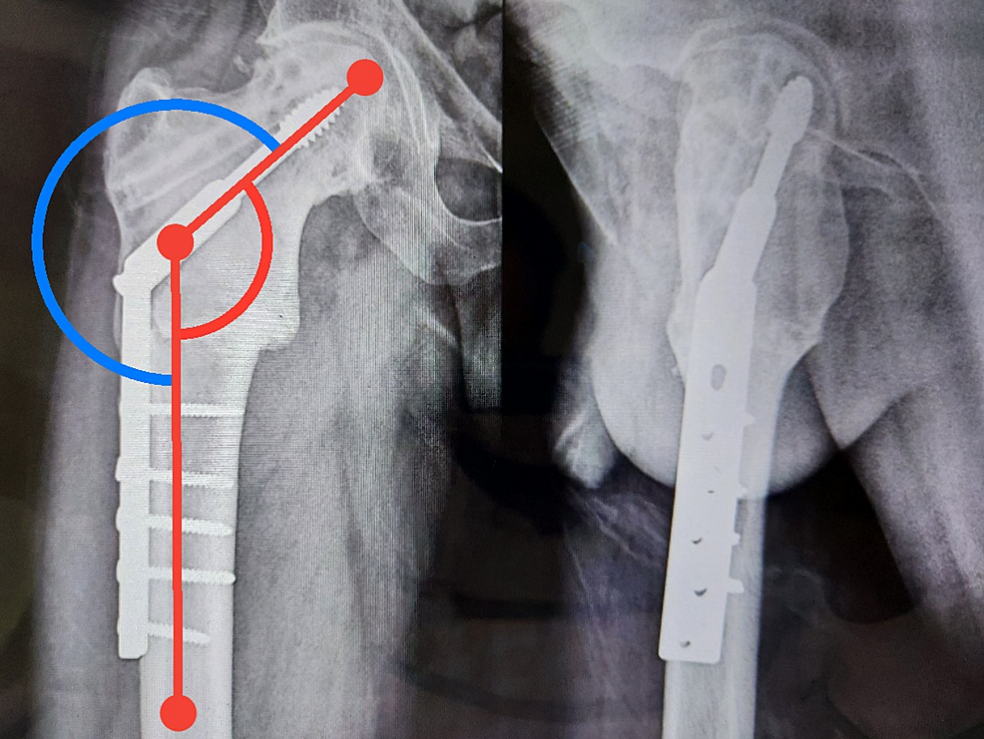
Follow-up x-ray after six months with the restored neck-shaft angle of 130 degrees
